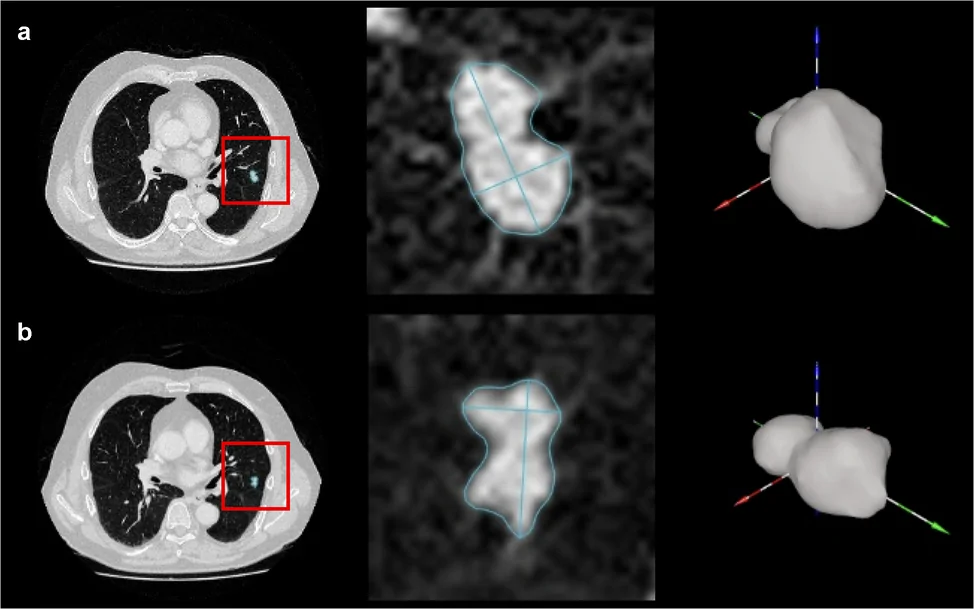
Axial non-contrast chest CT of an adult male patient, with AI-based pulmonary nodule detection (Veye Lung Nodules, version 3.26.3; DeepHealth, Amsterdam, NL) at diagnosis (a) and after 4 months (b). At diagnosis, AI annotation (*inside the red square*) highlights a solitary nodule in the left upper lobe, demonstrating an approximate 44% reduction in volume at response evaluation (courtesy of A.J. Hoving, MD, PhD, Department of Radiology, Spaarne Gasthuis, Haarlem, the Netherlands). *AI* artificial intelligence, *CT* computed tomography
